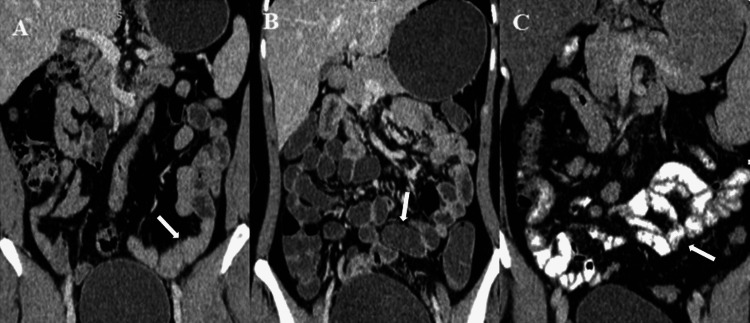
(A-C) Coronal reformatted CECT image showing bowel distension with oral plain water (A), bowel distension with mannitol (B), and bowel distension with positive oral contrast (C). Note the excellent bowel distension with mannitol when compared with water and positive iodinated contrast. CECT: contrast-enhanced computed tomography.
